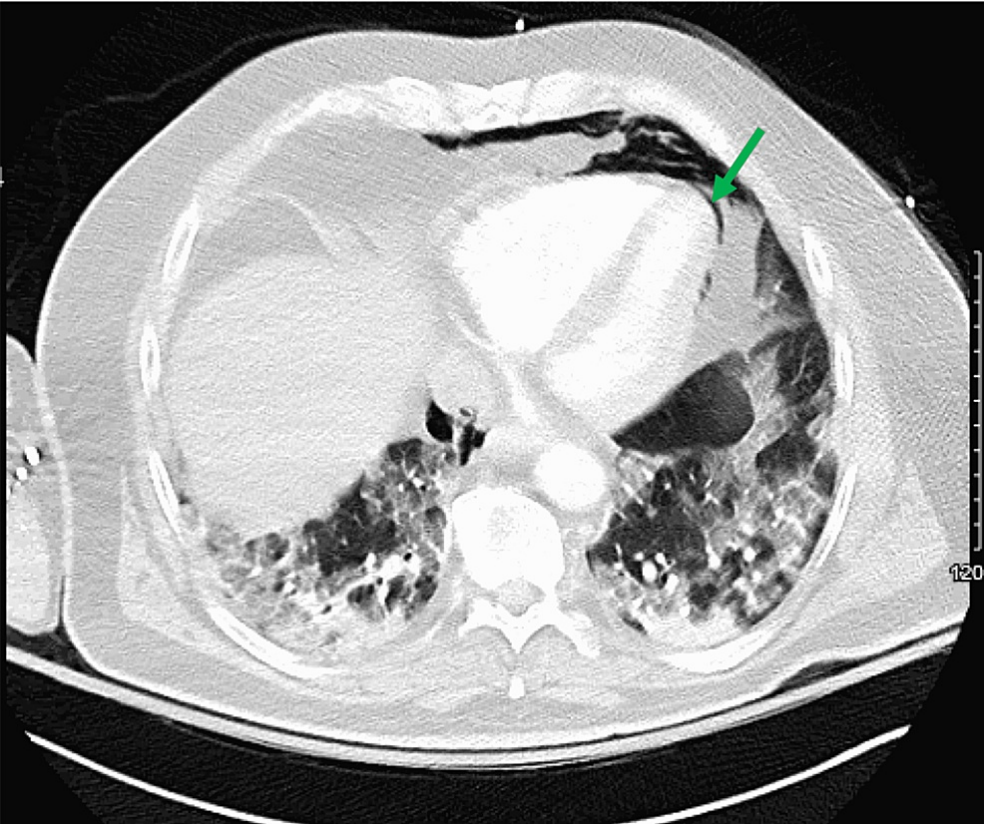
CT chest showing pneumopericardium (green arrow)
